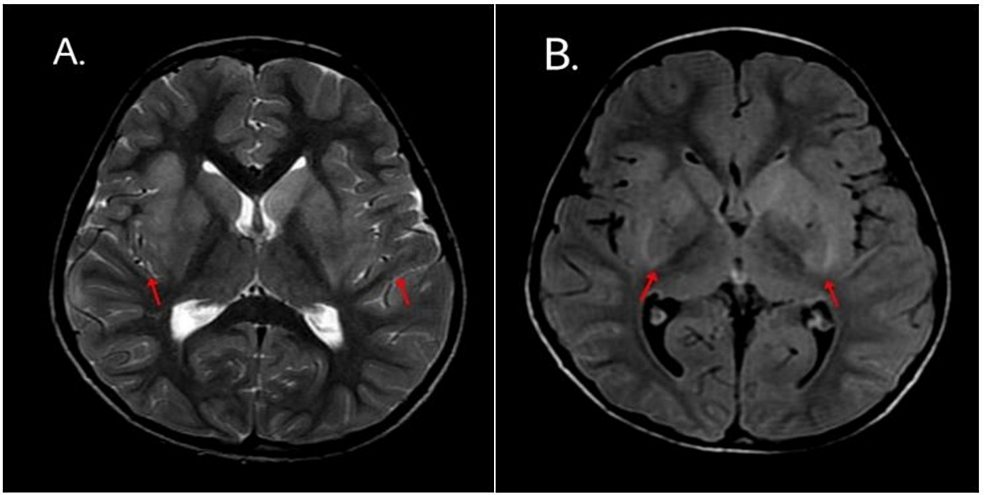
Axial (A) T2-weighted MRI and (B) FLAIR MRI of a six-year-old boy with COVID-19 encephalopathy show signs of symmetrical increase in signal intensity bilateral perisylvian cortex and bilateral basal ganglia suggestive of edema COVID-19: coronavirus disease 2019; FLAIR: fluid-attenuated inversion recovery
